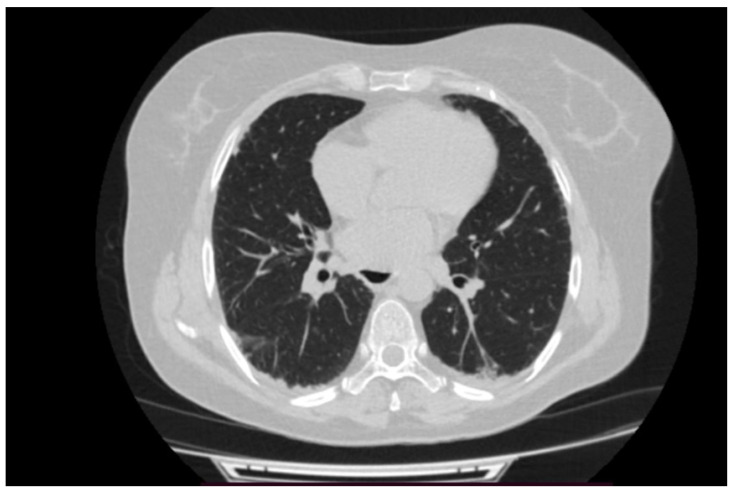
HRCT showing NSIP (transversal section).
